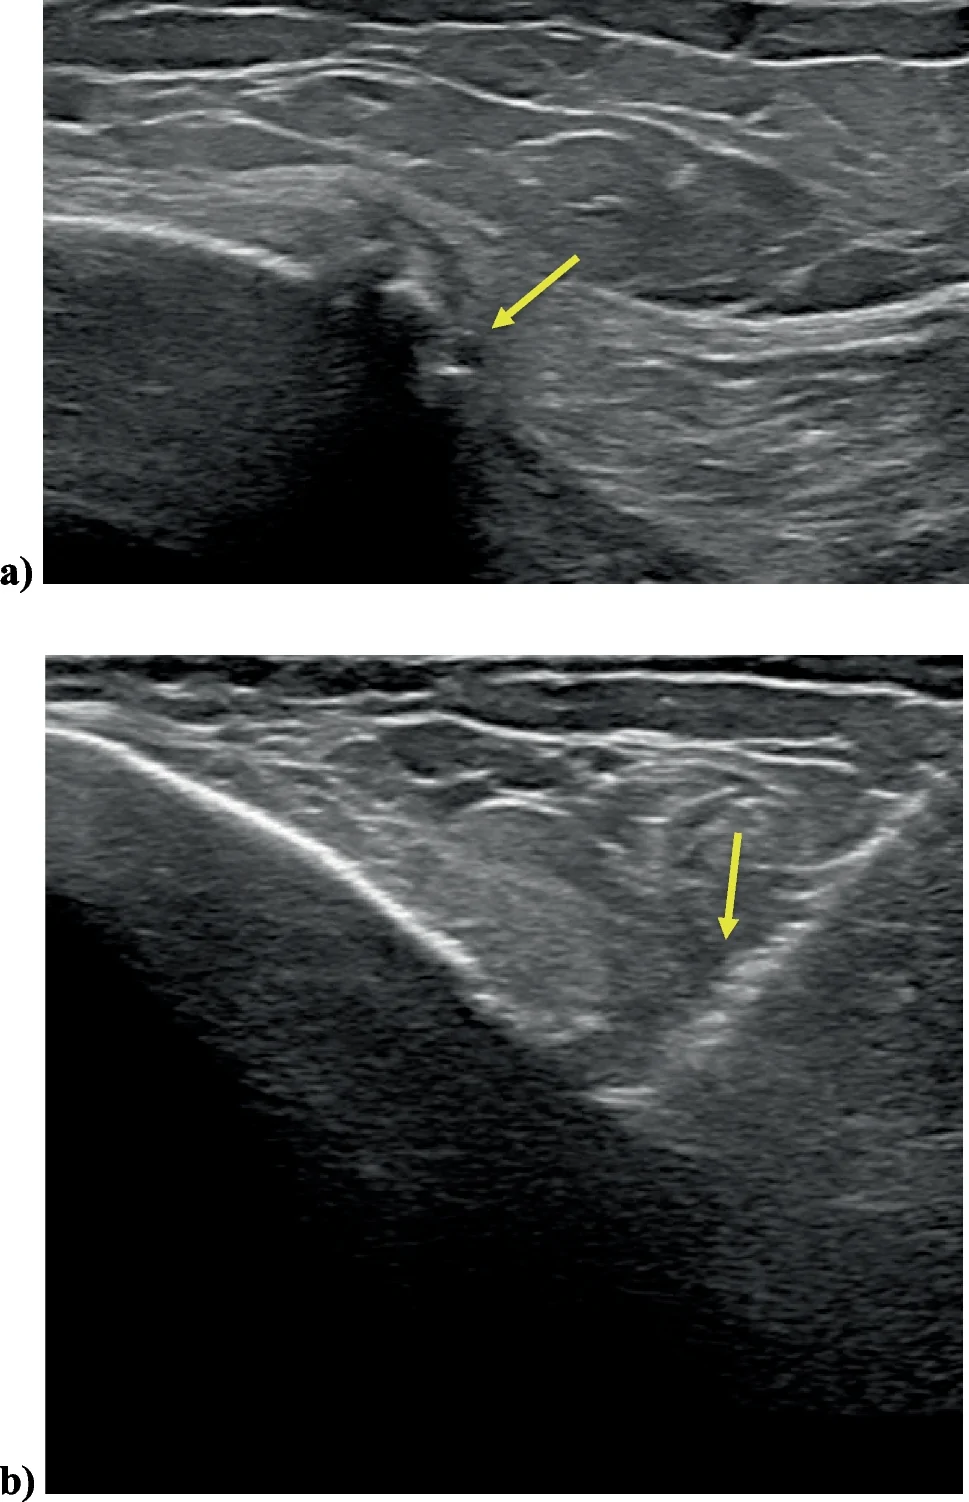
Ultrasound guided a localization of lesion and b needle advancement
